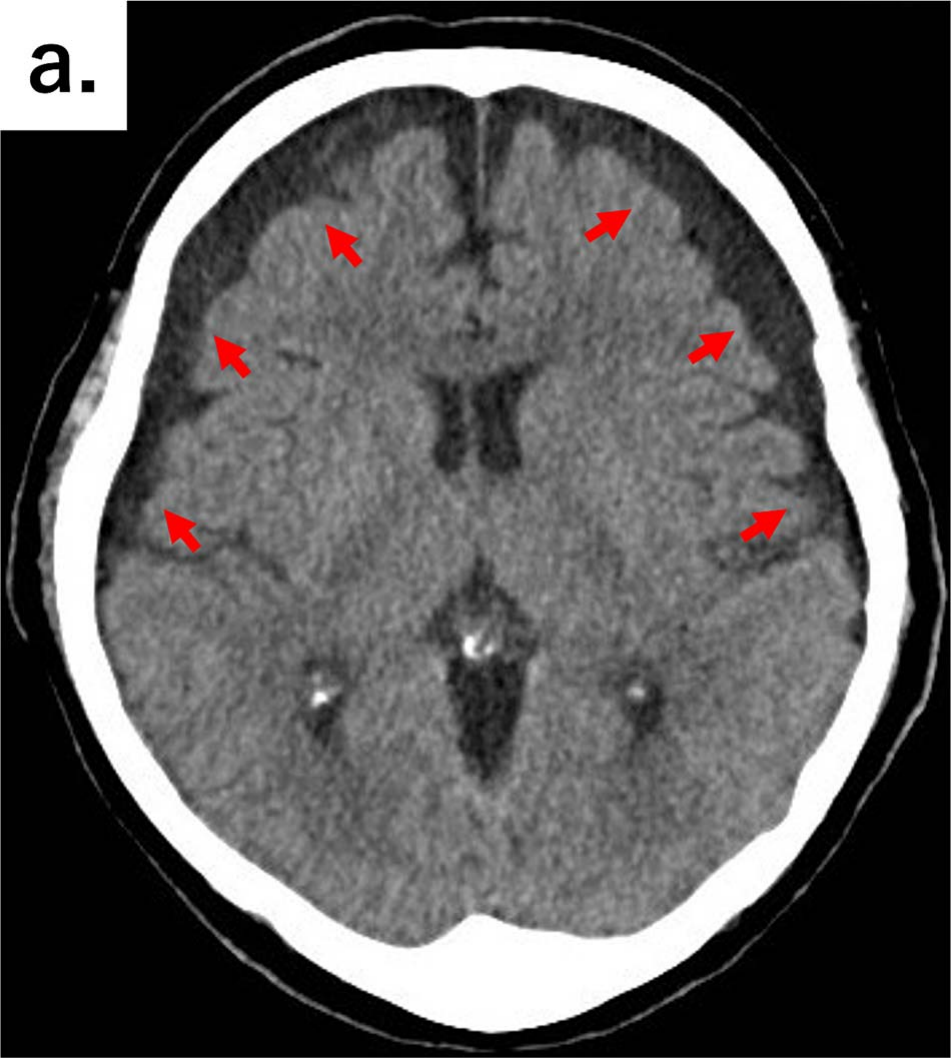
Axial CT image of the head showing bilateral chronic subdural hematomas (arrows).
